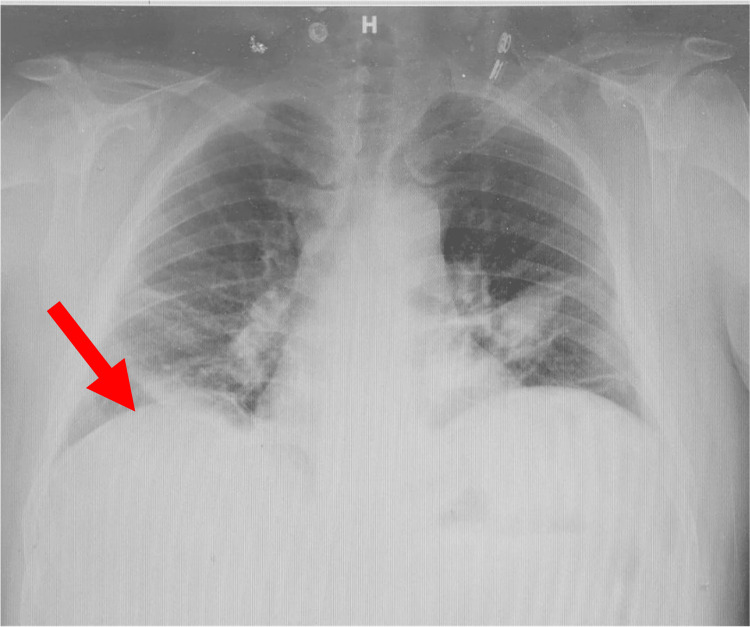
Chest X-ray at admission Admission chest radiograph demonstrating no free subdiaphragmatic air and clear lung fields bilaterally, excluding pneumoperitoneum.
